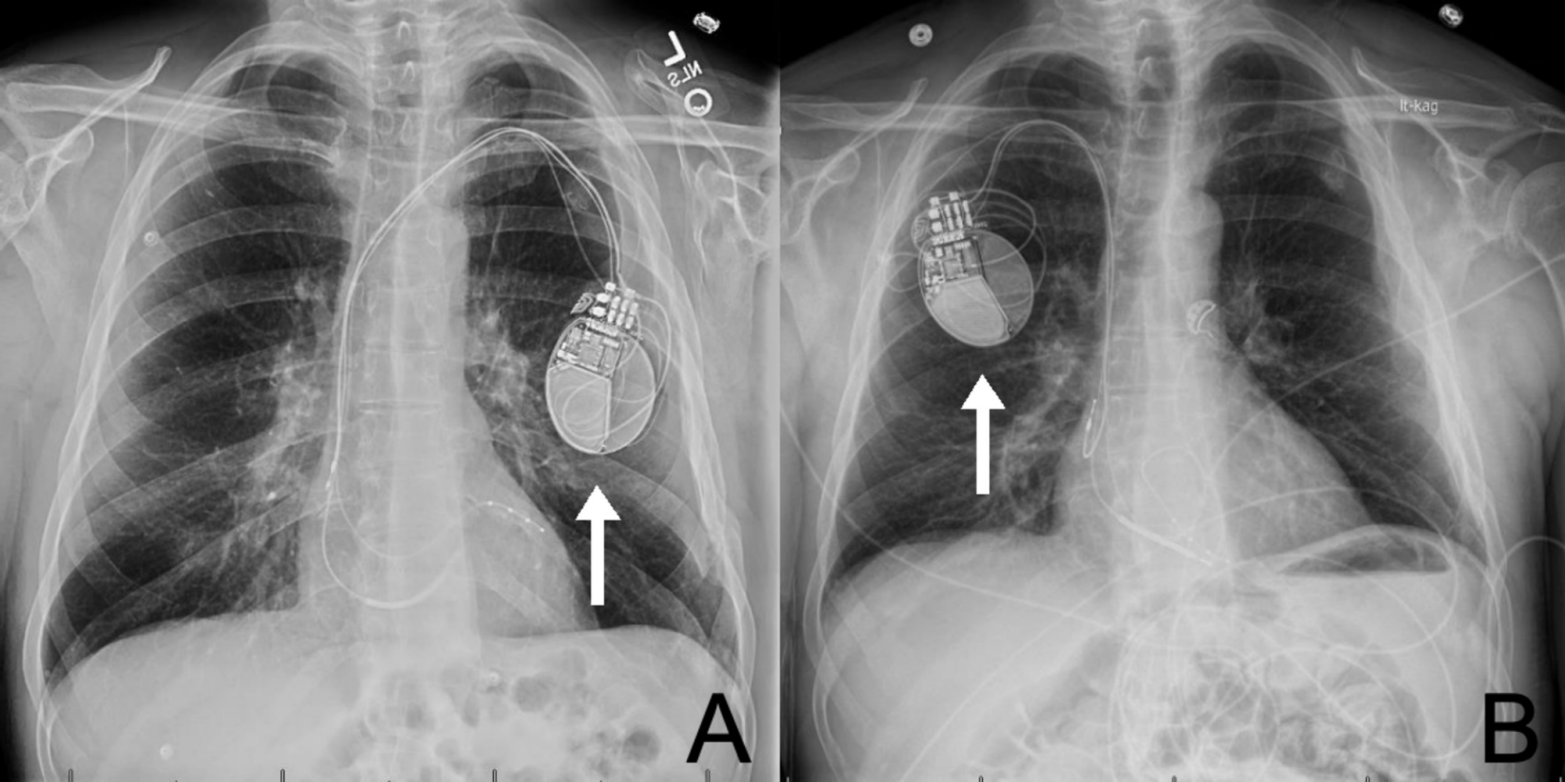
Chest Radiograph Anterior posterior chest radiograph of the patient detailing the original pacemaker site (A) and reinsertion site (B). Arrows are pointing at the pacemaker.
